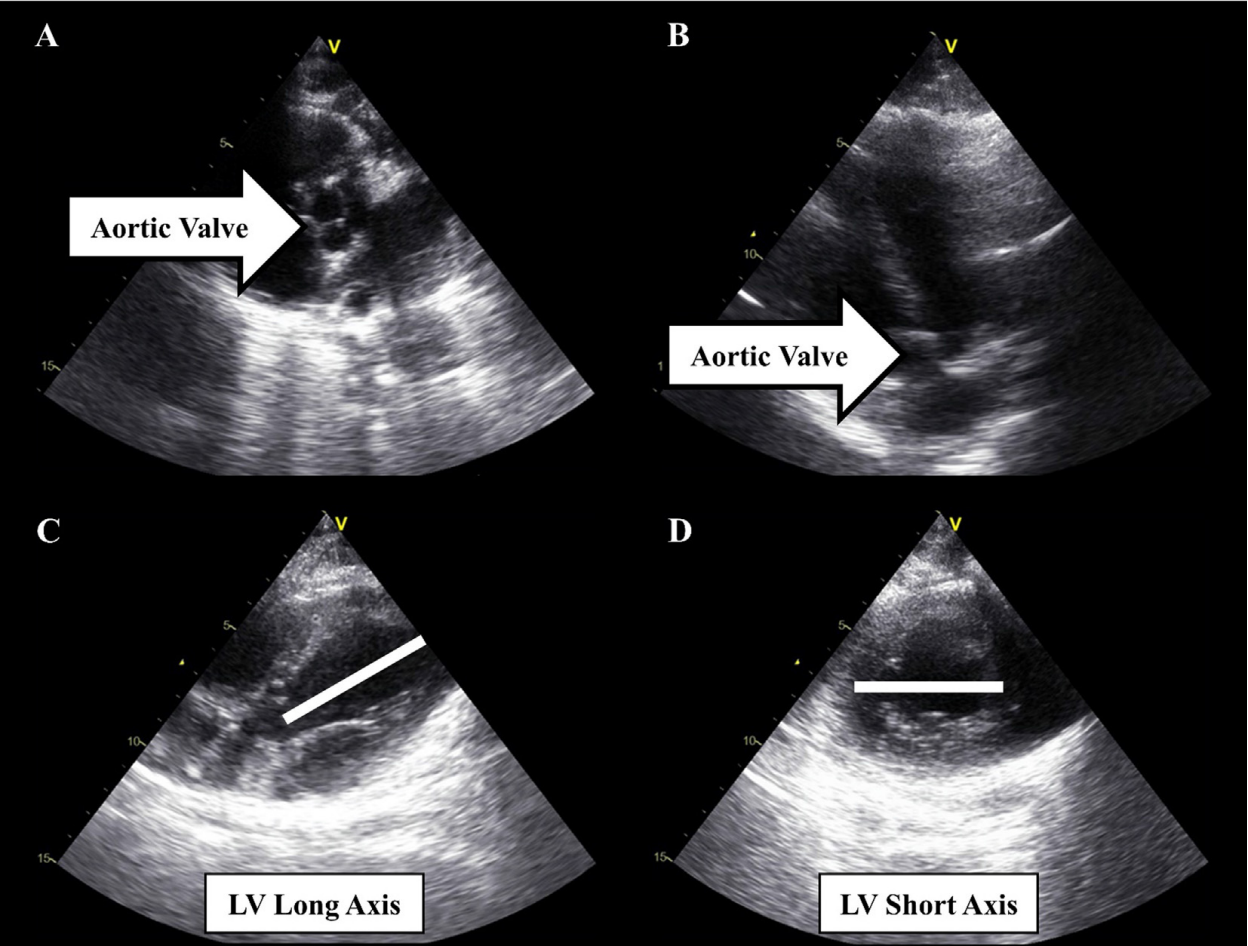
Transthoracic echocardiography 2-dimensional images of the aortic valve (A & B) to guide surface markings for the standard chest compression (SCC) location. Left ventricular long (C) and short (D) axes used to guide surface markings for the left ventricle chest compression (LVCC) location. The white lines in C and D indicate the corresponding axes.
